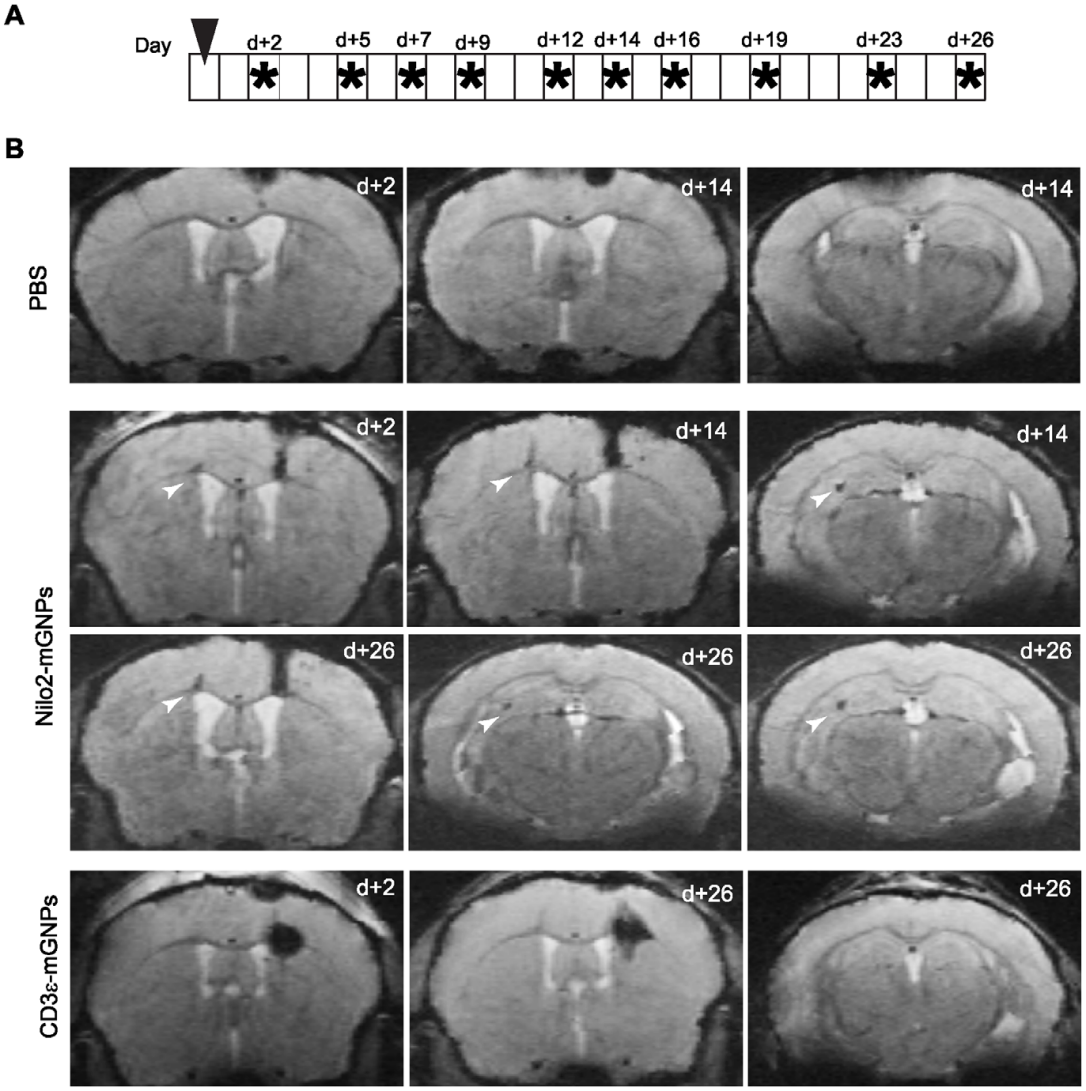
Nilo2-mGNPs identify neuroblasts in the main neurogenic niches. A , Experimental schedule, Nilo2-mGNPs injection day is indicated with an arrowhead, MRI acquisition days are shown with asterisks. B, Axial views at different rostrocaudal positions of a representative MRI at different time points after the injection of PBS (1 µl, n = 2), Nilo2-mGNPs (n = 2) or CD3ε-mGNPs (n = 2) into the right hemisphere in the absence of tumor induction. Arrowheads indicate the hypointense signals in neuroblasts niches.
